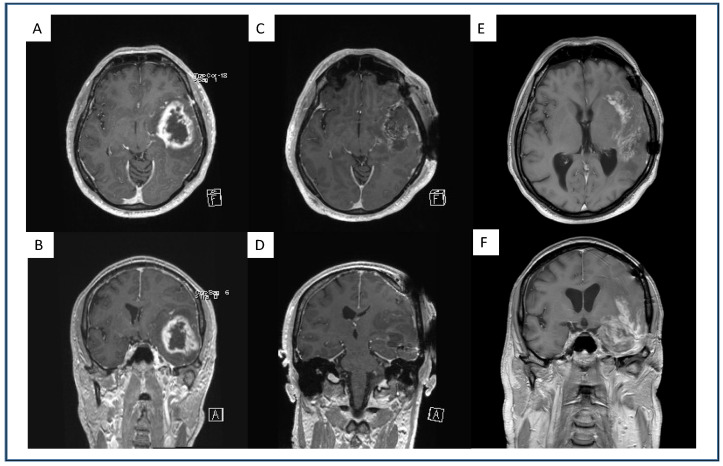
Exemplary representation of a dull demarcated GBM under therapeutic AC, on the first recurrence after standard-of-care treatment. Preoperative axial (A) and coronal (B) gadolinium-enhanced, T1-weighted MRI of a GBM patient with a left fronto-temporal contrast-enhancing lesion. Postoperative axial (C) and coronal (D) gadolinium-enhanced, T1-weighted MRI of the same patient after GTR where no contrast enhancement is present. Axial (E) and coronal (F) gadolinium-enhanced, T1-weighted MRI of the same patient with therapeutic AC upon first progression with a dull demarcated recurrent lesion.
